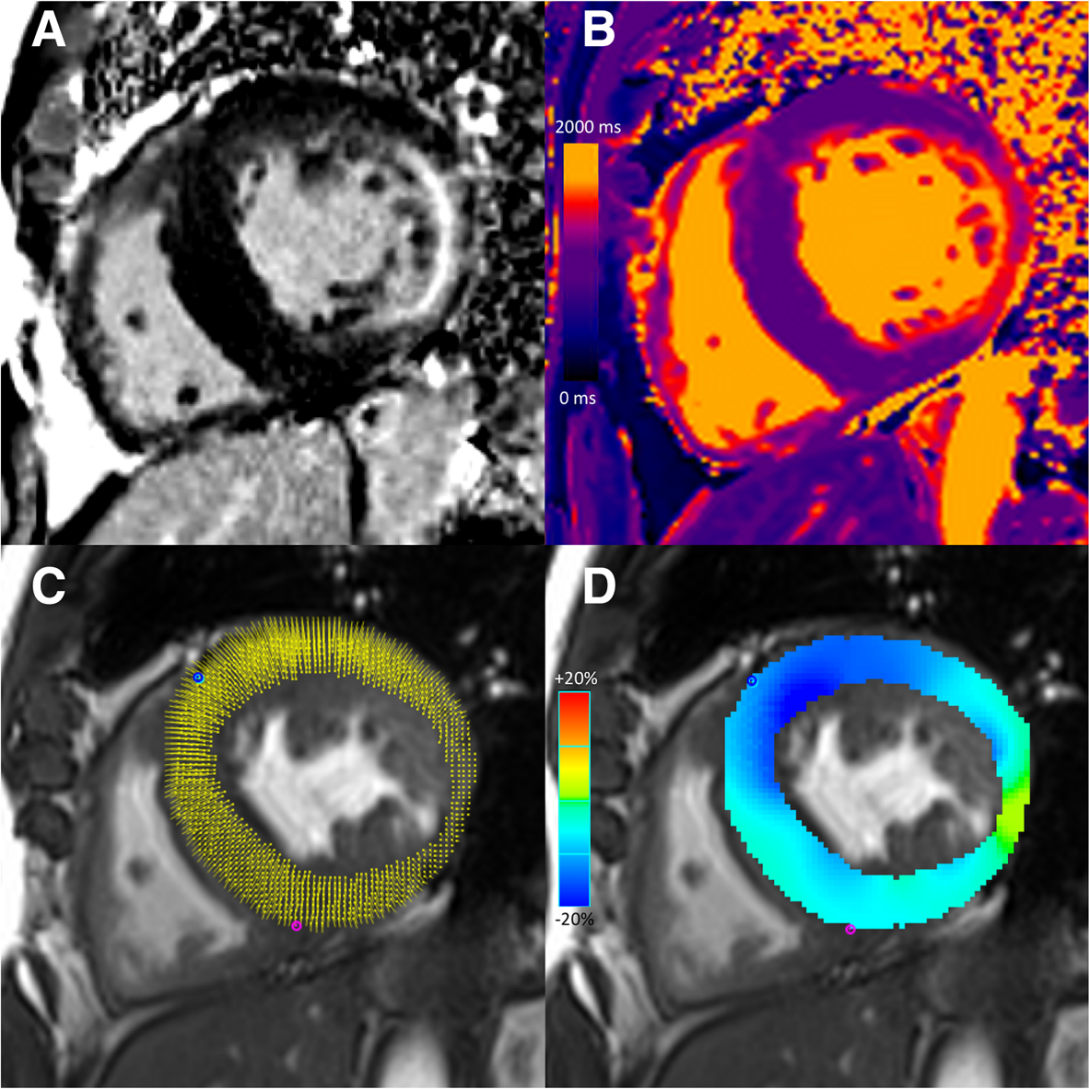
Cardiovascular magnetic resonance images of a 57-year-old male with Fabry disease. Short-axis late gadolinium enhanced (LGE) image demonstrates concentric left ventricular hypertrophy and mid-wall LGE at the inferior lateral wall (a). Short-axis native T1 map (native T1 value 1100 ms at the interventricular septum) (b). Short-axis cine balanced steady state free precession (bSSFP) image with circumferential myocardial strain analysis points (c) and color myocardial circumferential strain map (d). Global longitudinal strain was − 10.4%. Global circumferential strain was − 15.0%. Base-to-apex longitudinal strain gradient was 2.6%. Base-to-apex circumferential strain gradient was 2.1%
